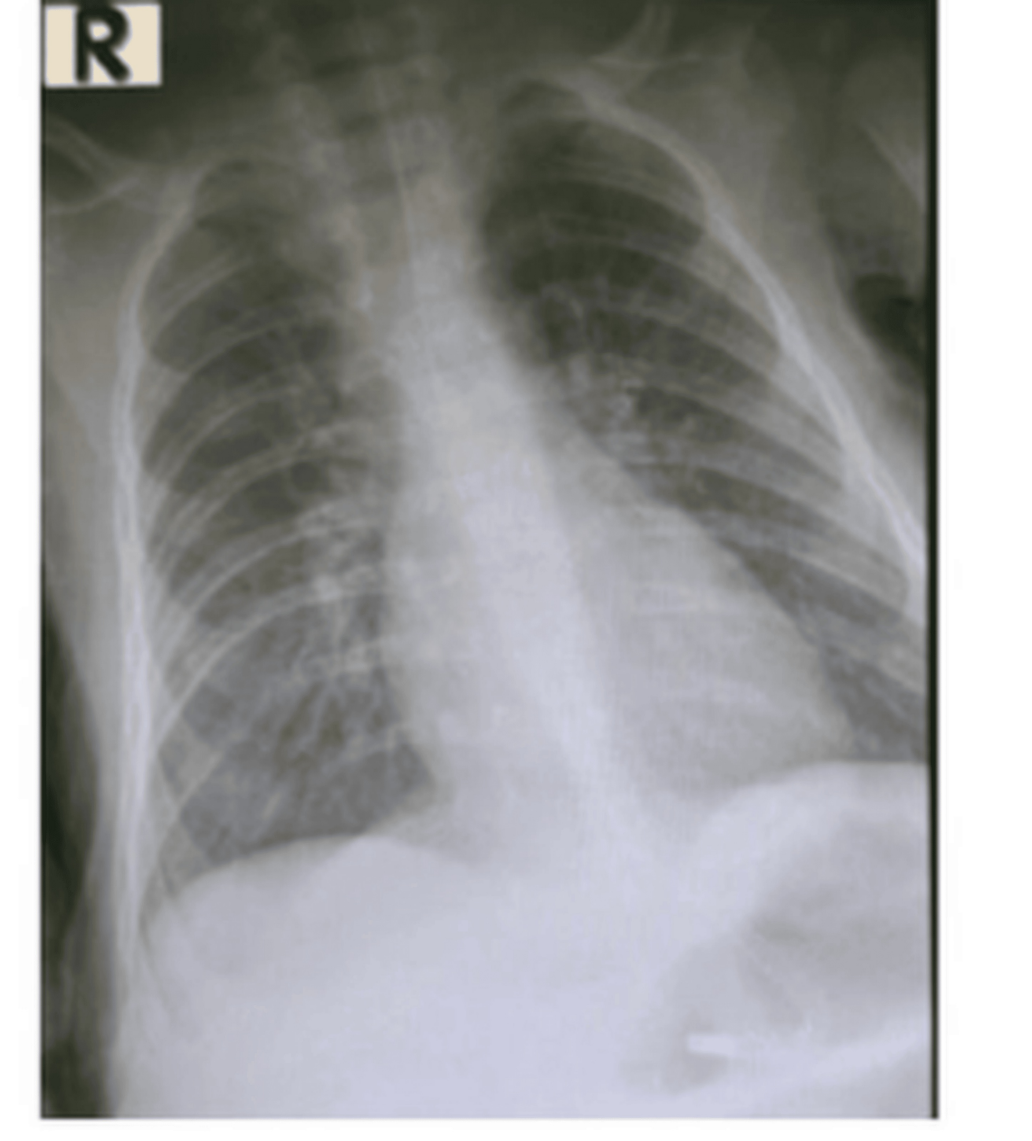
Chest radiograph of the infant with malignant pertussis The image demonstrates diffuse bilateral perihilar infiltrates and hyperinflation.
